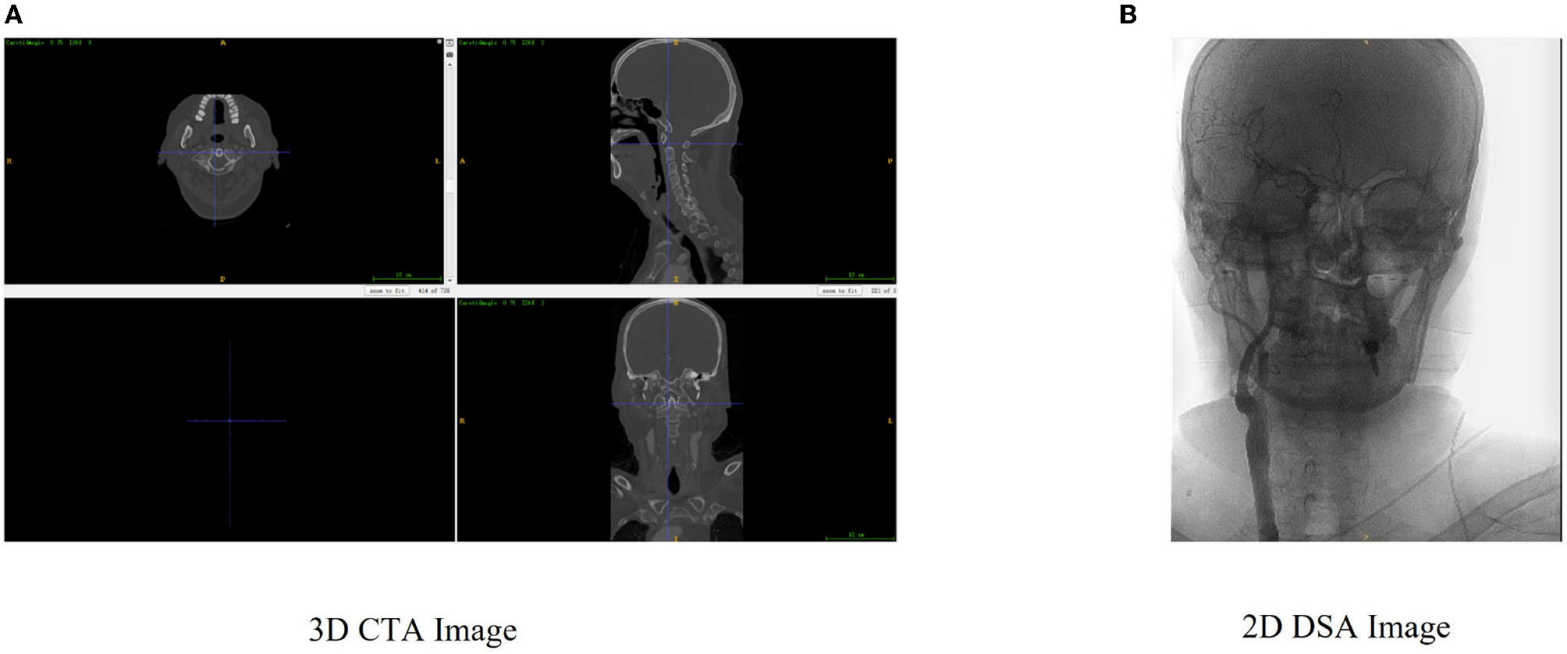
A comparison of three-dimensional computed tomography angiography (3D CTA) image and two-dimensional digital subtraction angiography (2D DSA) image presentation, where (A) 3D CTA image is a floating image, and (B) 2D DSA image is the reference image.
